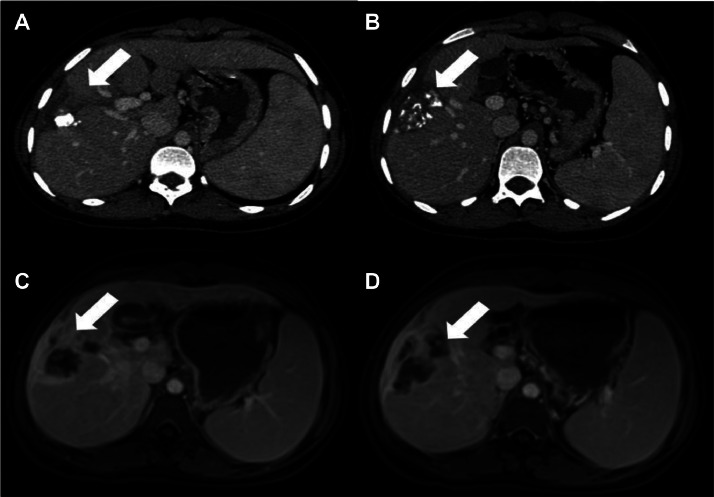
Post first TACE, axial CT scan (A, B) axial injected T1 (C, D) showing a dense and heterogeneous Lipiodol retention in treated lesions.
